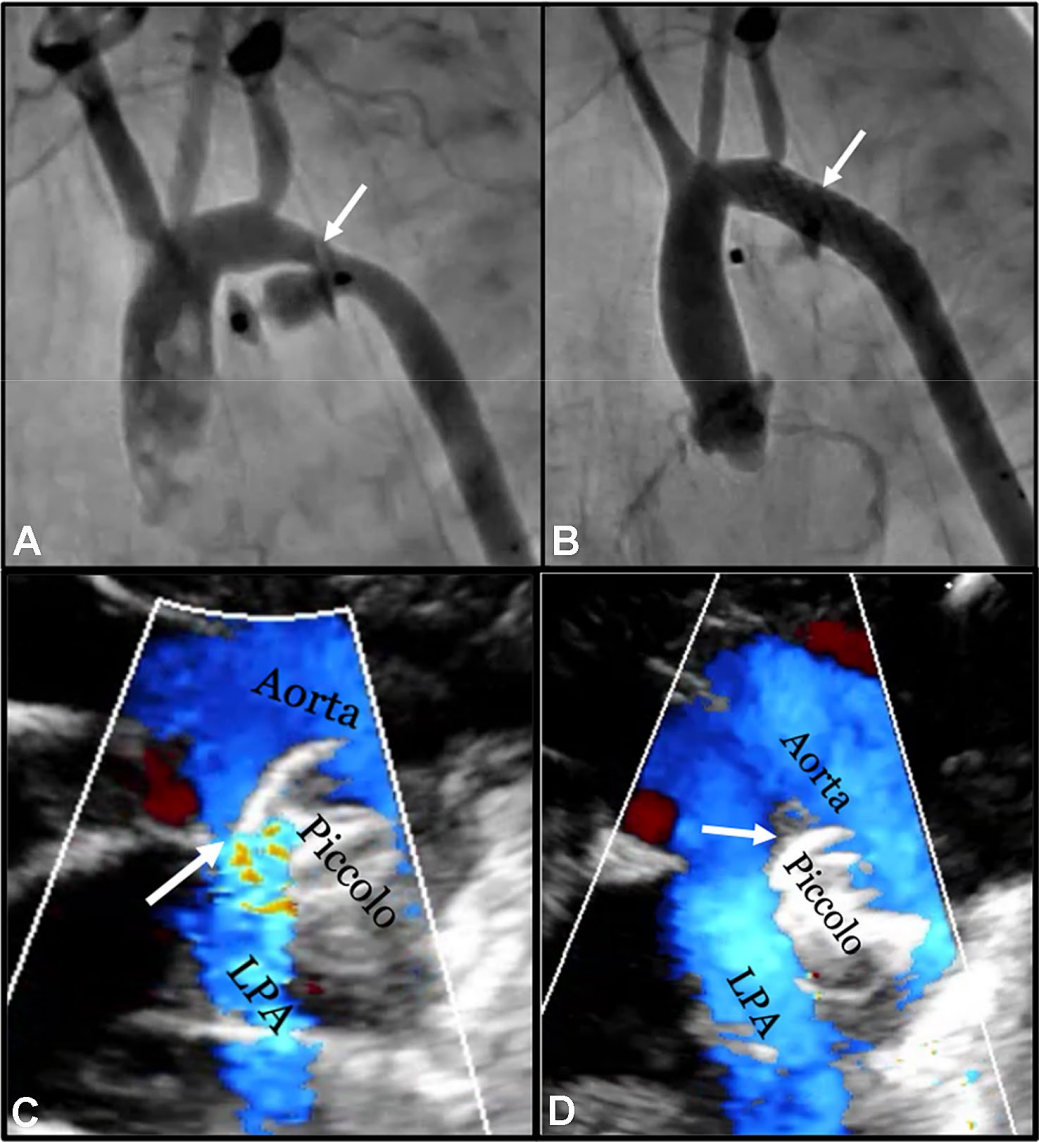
Device Protrusion Causing Aortic or Left Pulmonary Artery Obstruction. A Extraductal implantation with distal disc projecting into the aorta leading to aortic arch obstruction (ARROW) in a 540-g infant. B Aortic arch obstruction in the infant (A) treated with stent implantation from a carotid approach with no residual stenosis (ARROW). C Extraductal implantation with proximal disc projecting into the LPA (ARROW) leading to LPA stenosis in an 800-g infant (echocardiographic parasternal ductal view). D Intraductal repositioning of the device (ARROW) in the infant (C) with disc no longer causing LPA stenosis
